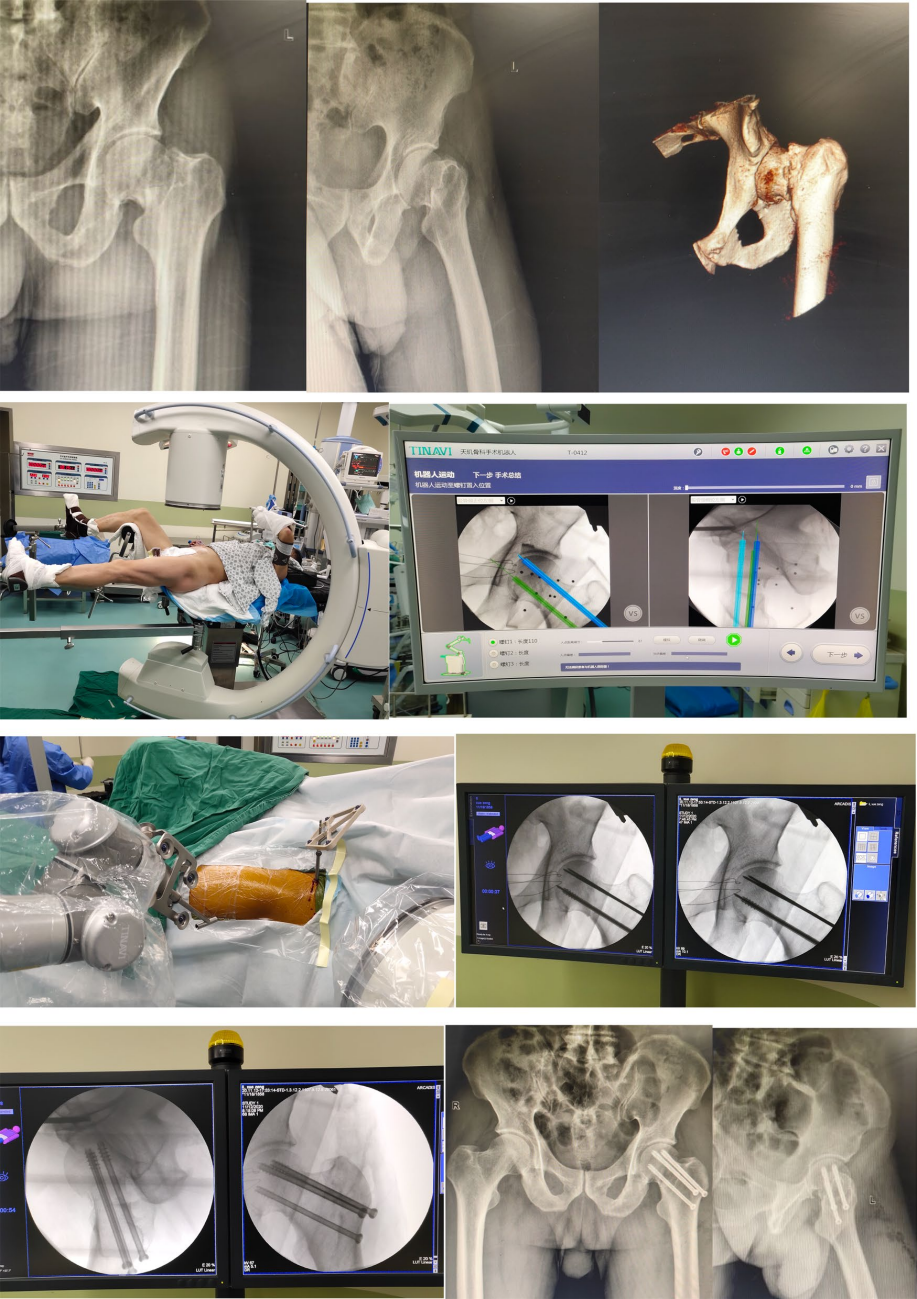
Patient 2, male, 61 years old, a, b X-ray showed: left femoral neck fracture, Garden type III. c The affected limb was first placed in traction until the alignment of the femoral neck fracture was satisfactory, and then routine lower limb disinfection was performed. d, e The robot's computer plans the path, length, and diameter of the femoral neck hollow nail placement. f–h Then, the surgical robot is run to position the guide cannula in the planned position, and three parallel guide pins are drilled in sequence, with the position, direction, and length of the guide pins again fluoroscopically appropriate. i Finally, the hollow drill bit is drilled through the lateral femoral bone cortex with the guide pins, and the 7.3-mm diameter hollow screw is selected for placement with the guide pins, and the screw is tightened with gradual pressure. j, k Postoperative X-ray fluoroscopy showed satisfactory fracture repositioning and hollow screw position
